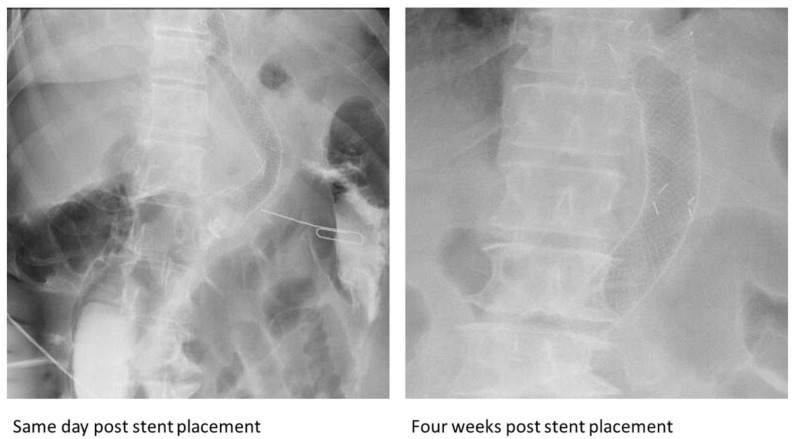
Subsequent radiographs after the placement of the esophageal self-expandable metal stent in the third case, demonstrating acute angulation in the distal third at a nearby location of the partial fracture.
